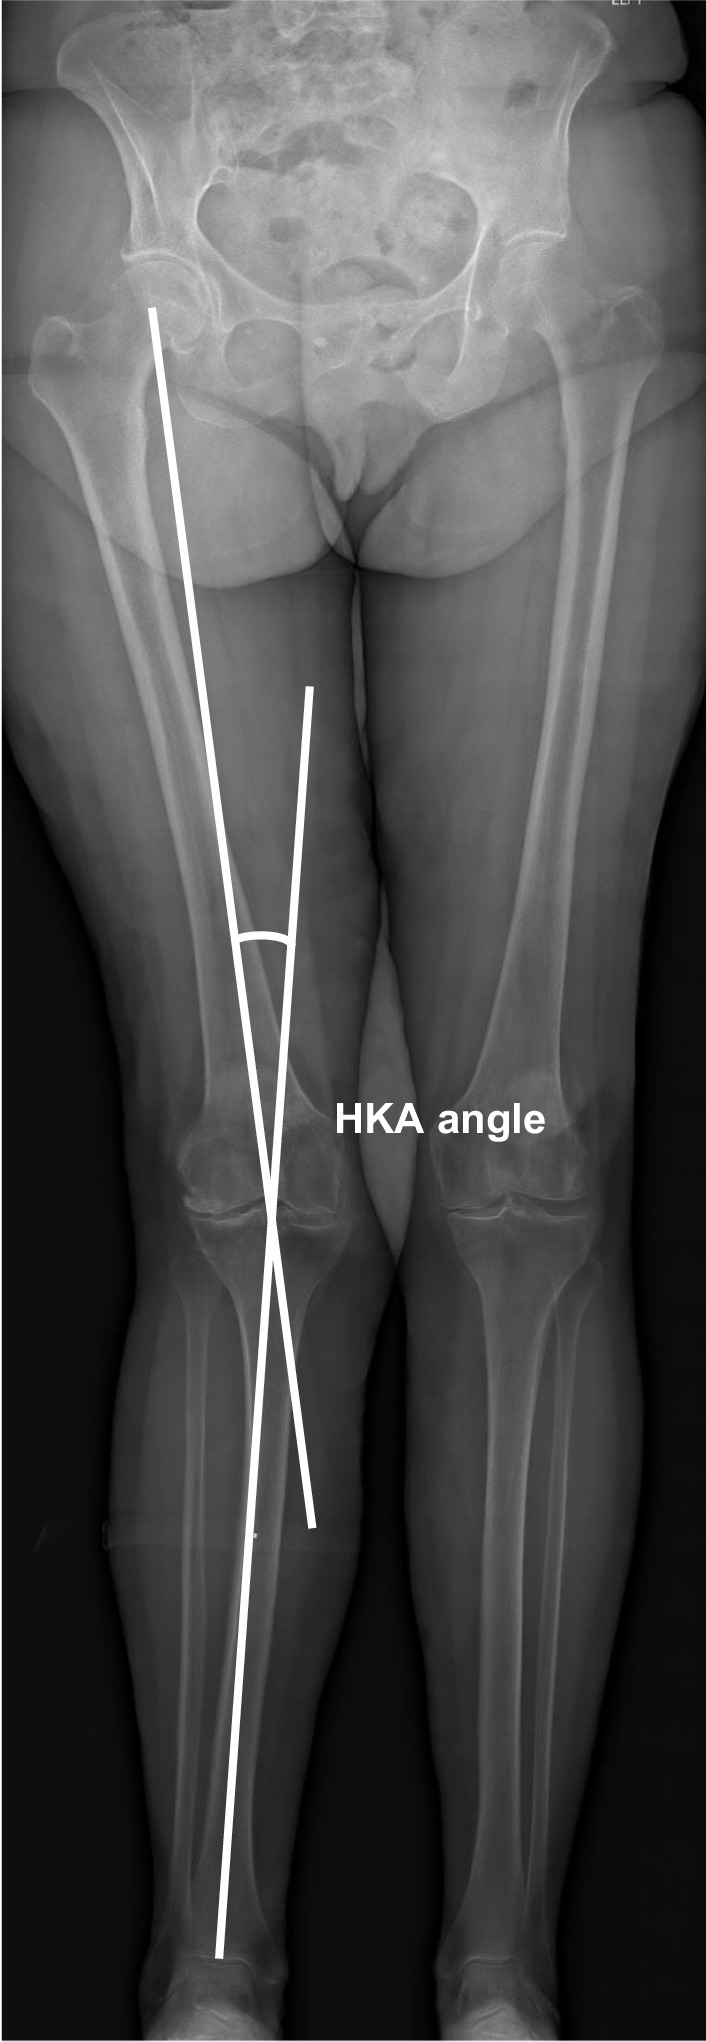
Hip–knee–ankle (HKA) angle. A pre-operative weightbearing lower-limb anteroposterior EOS digital radiograph is shown. The HKA is the angle between the femoral and tibial mechanical axes. Varus, negative; valgus, positive
